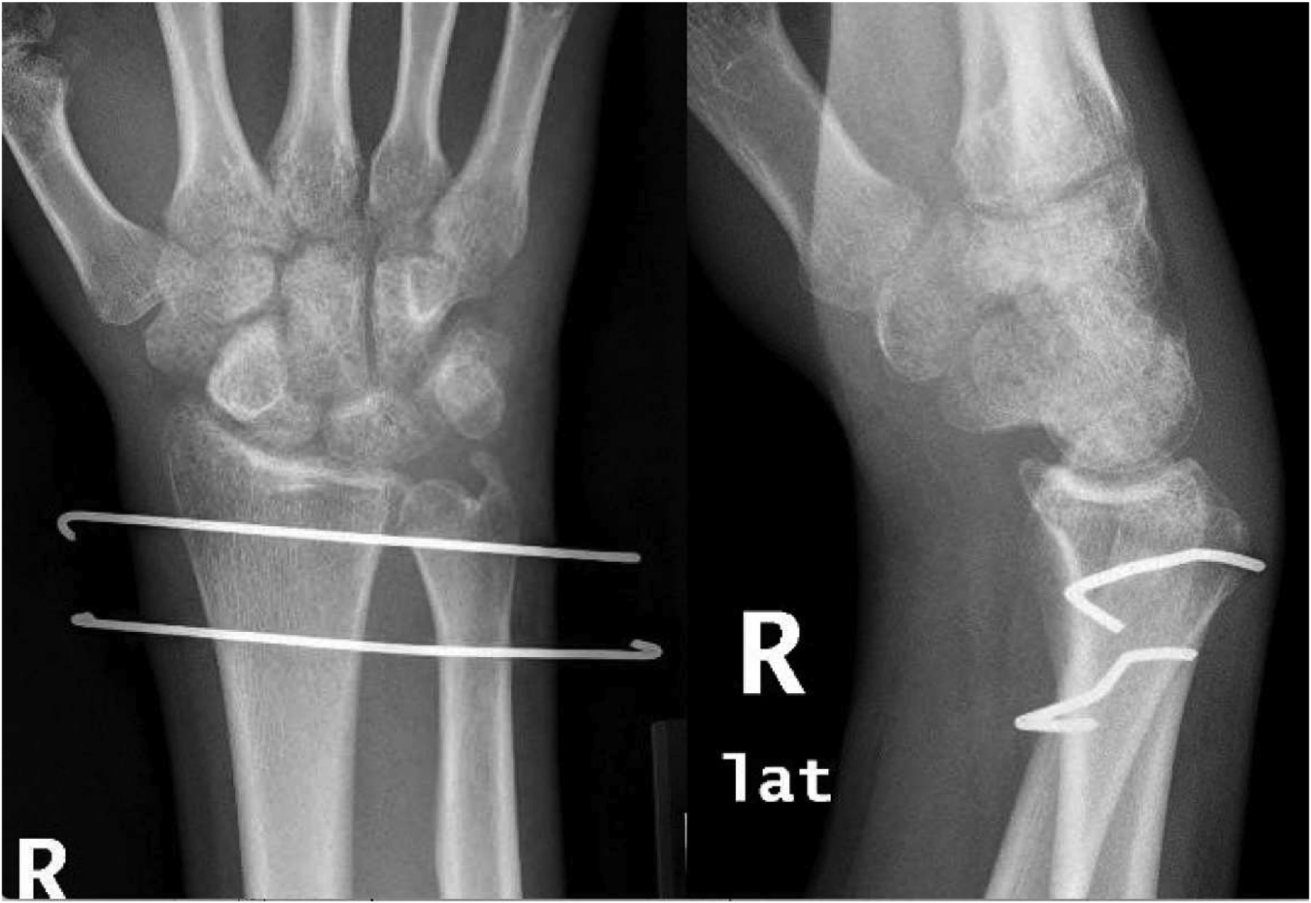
Radiographs of the wrist after reduction.
